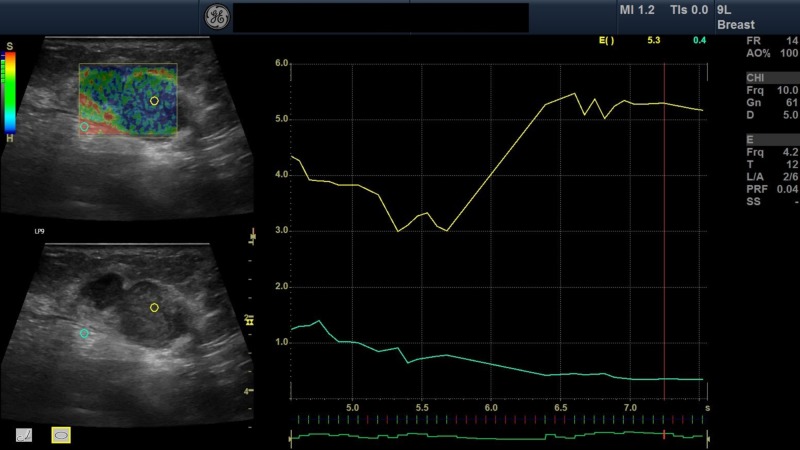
Elastography image of breast lesion A 43-year-old woman presented with concerns of left side breast pain and mass in the lower outer quadrant that was firm in consistency on palpation. The mass was non-mobile with no discharge. On B-mode ultrasonography followed by strain elastography, the mass was well-defined rounded heteroechoic with minimal vascularity and no area of calcification. Mass was graded as BI-RADS grade 3 followed by strain elastography, two regions of interest (green and yellow) were taken. The green and yellow regions of interest represent the normal area and breast mass, respectively, with their graphic representation on the left side. The elastography score of the lesion was 2, and the strain ratio was 1.2.
